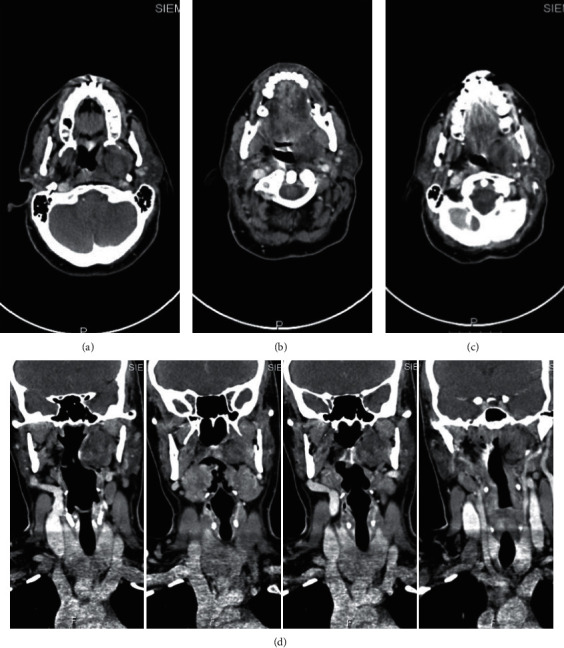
(a) Axial cut CT images revealing left-sided PPS. (b, c) Coronal cut CT images showing the PPS arising from the deep lobe of the left parotid. (d) Minimal mass effect of the tumor on the oropharyngeal airway.
